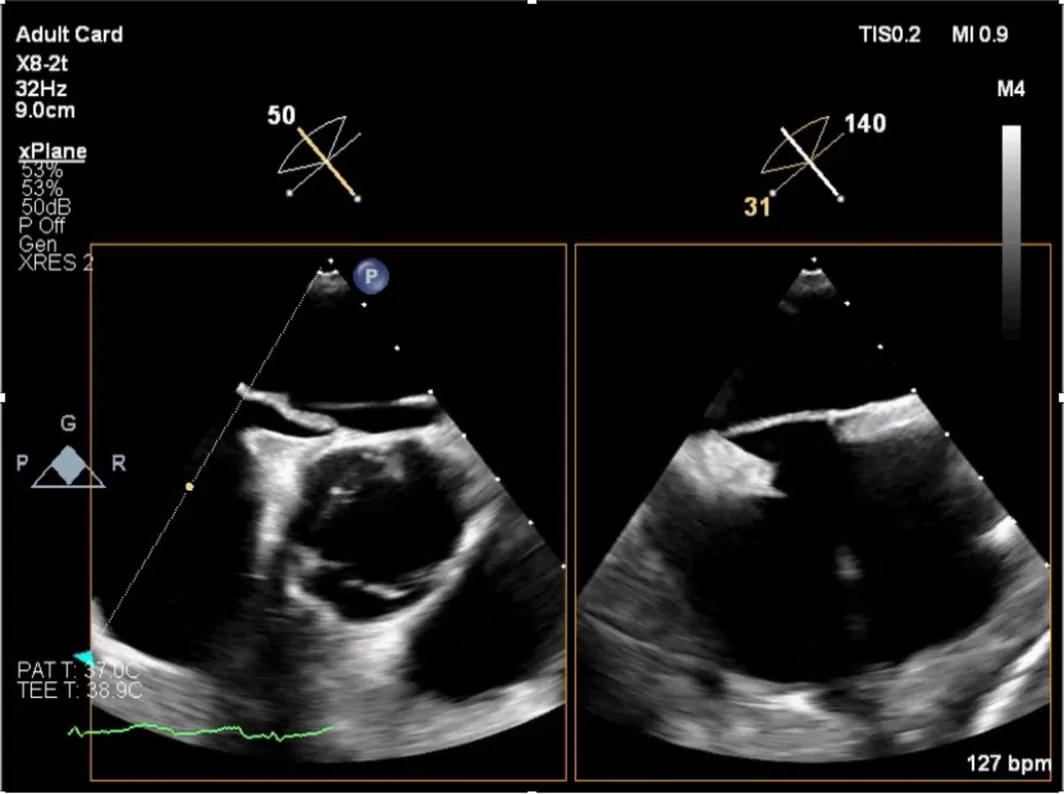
Transesophageal biplane view during passing the wire through patent foramen ovale.
